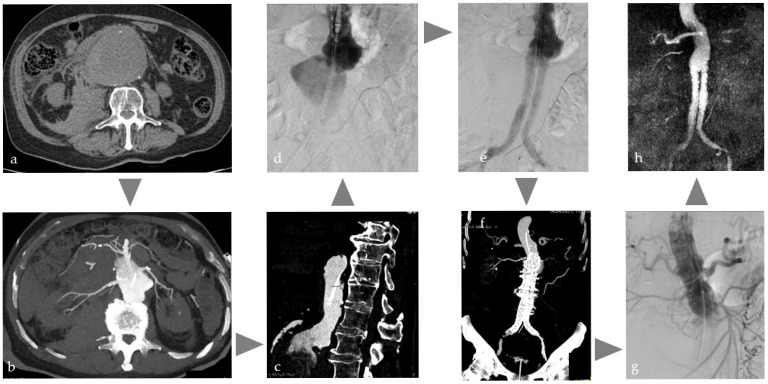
Typical workflow for one-stop treatment of a partially ruptured abdominal aortic aneurysm in a patient with acute abdominal pain and restricted kidney function: (a) Due to clinical and sonographic suspicion of a ruptured abdominal aortic aneurysm, native CT on the Angio table to confirm findings, (b,c) CT with intra-arterial injection of 120 mL volume, with a dilution of 1:5 corresponding to approx. 20 mL CM for planning the EVAR, (d) insertion of the main body of the prosthesis, (e) control DSA after bi-iliac stent graft with indication of insufficient coverage and type 1a endoleak, (f) immediate intraoperative CT control with intra-arterial injection as above to plan prosthesis extension, (g) treatment using cuff in chimney technique, (h) MRI control the next day with regular findings.
